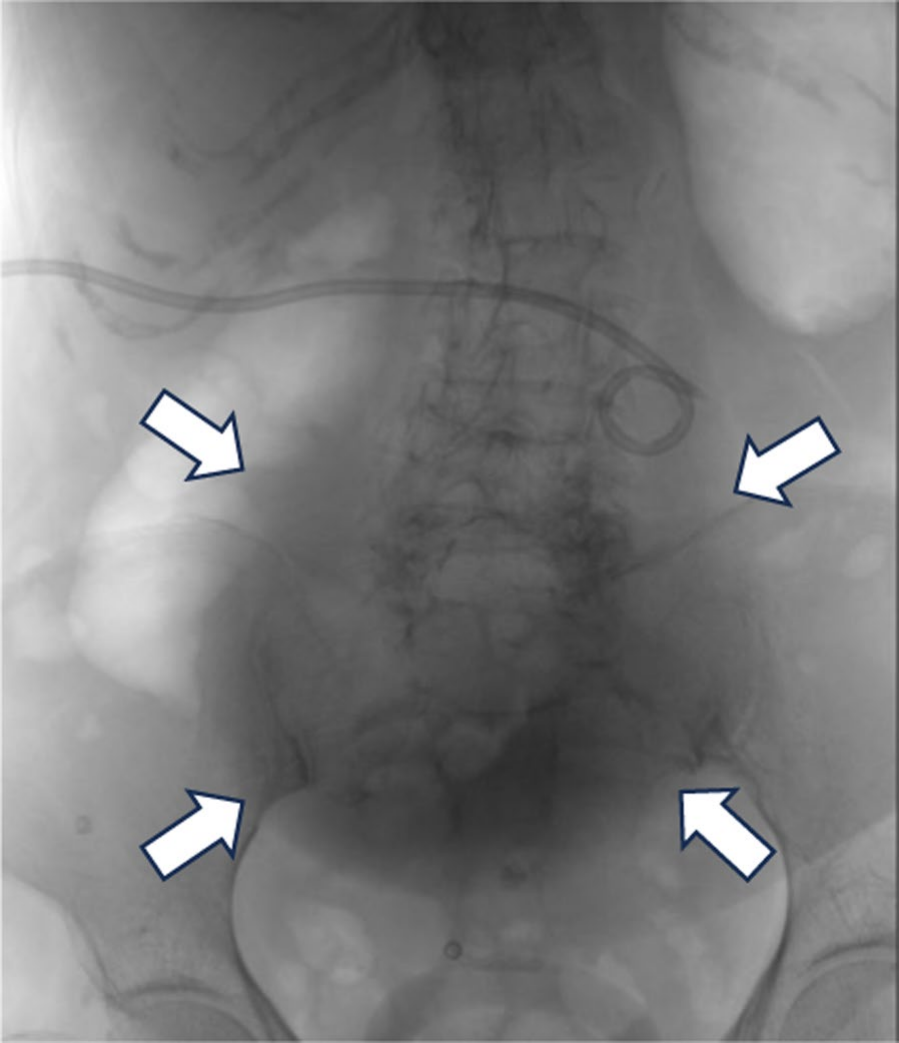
X-ray photograph showed the cavity after PTGBD insertion (white arrows)
